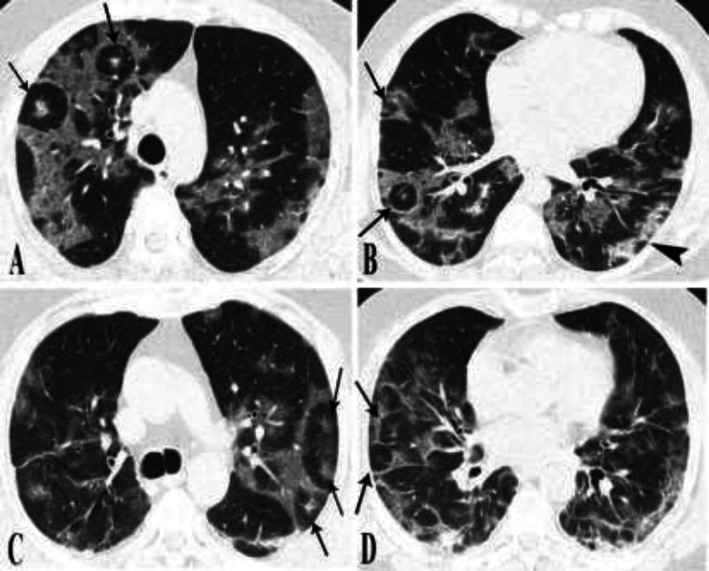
Chest CT scans of cases 3-4. (A, B) case 3. CT scan shows several foci of target shaped lesions (arrows). Arrowhead shows parenchymal band indicating the later phases of COVID-19 induced pneumonia. (C, D)
